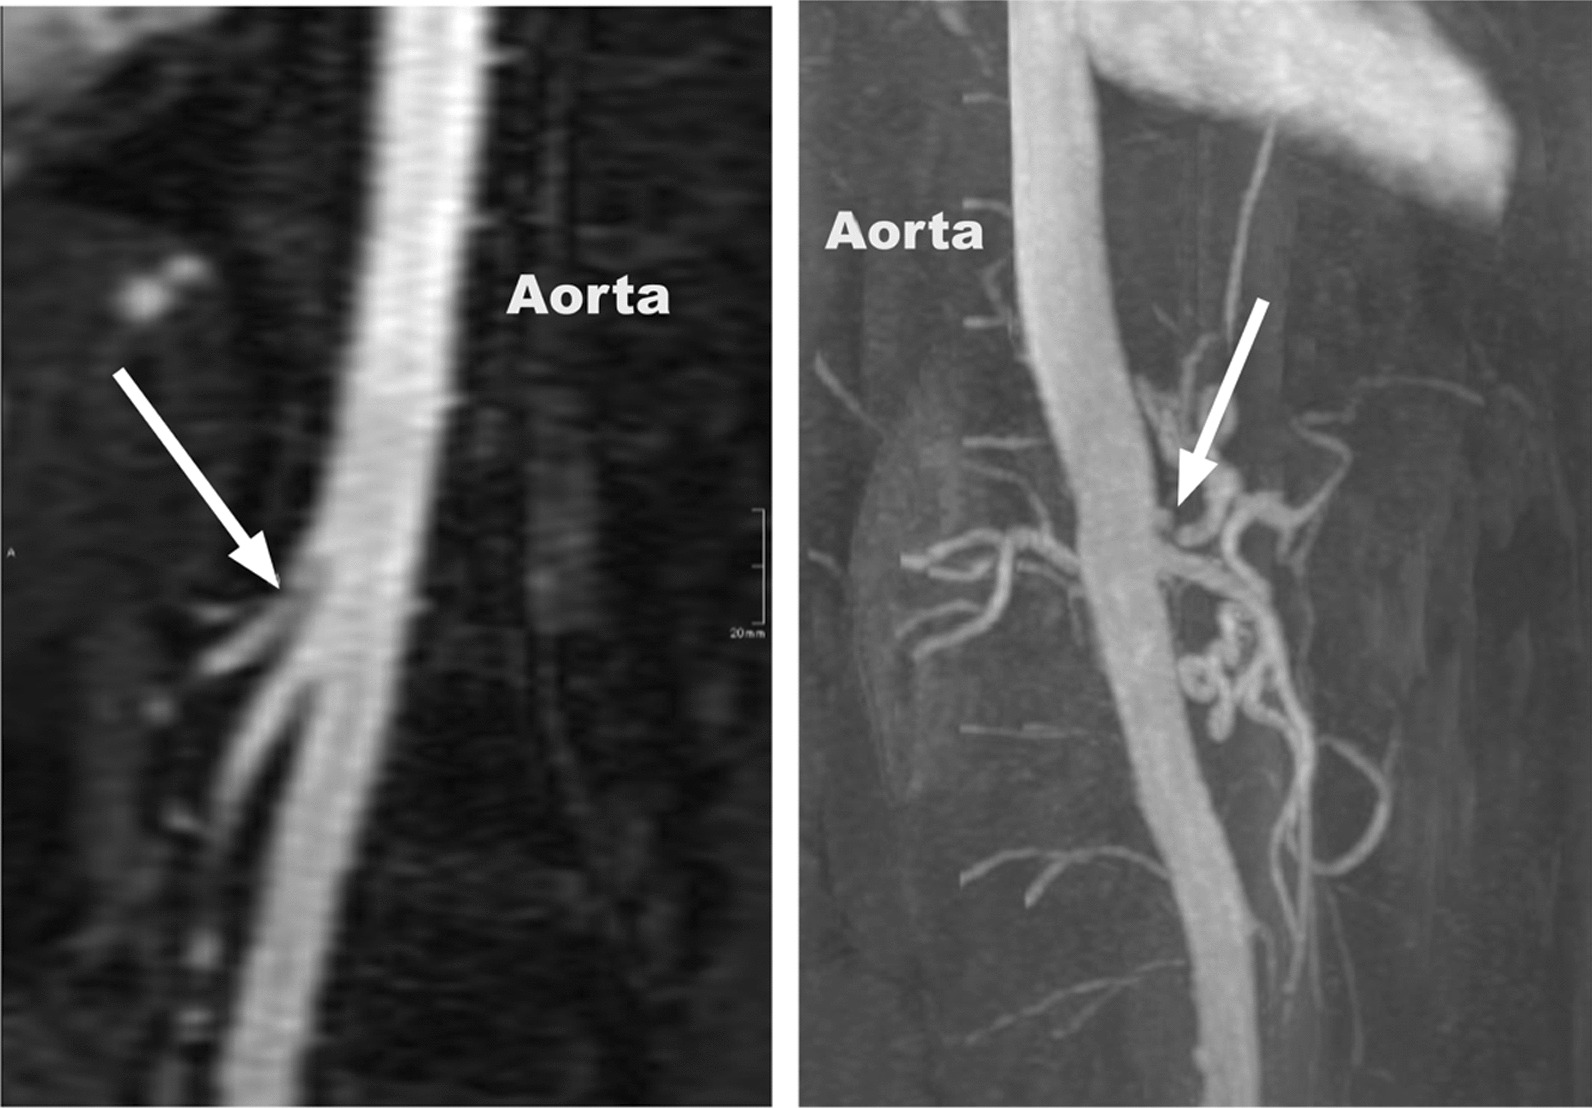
Computed tomography angiography (CTA) image (left) and 3D MRI reconstruction (right) showing celiac artery stenosis
